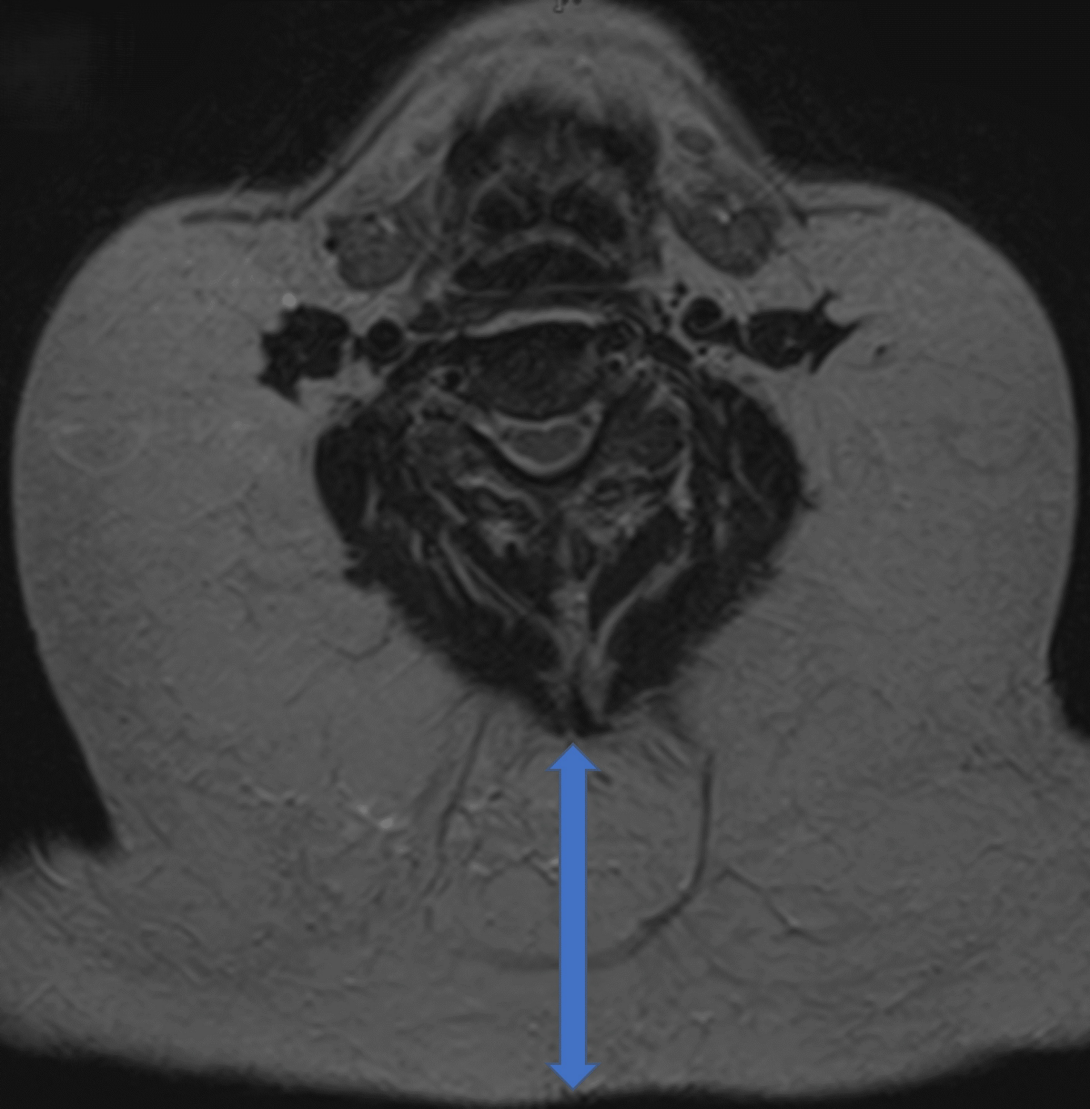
MRI imaging at recurrence T1-weighted axial image showing circumferential cervical adipose proliferation measuring 70 mm at the C4/5 vertebral level with mild airway compression.
